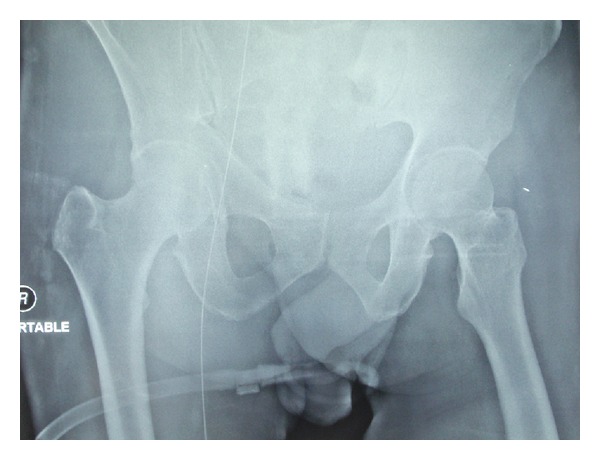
Pelvic X-ray showing the guidewire extending into the femoral vein in the right thigh.
